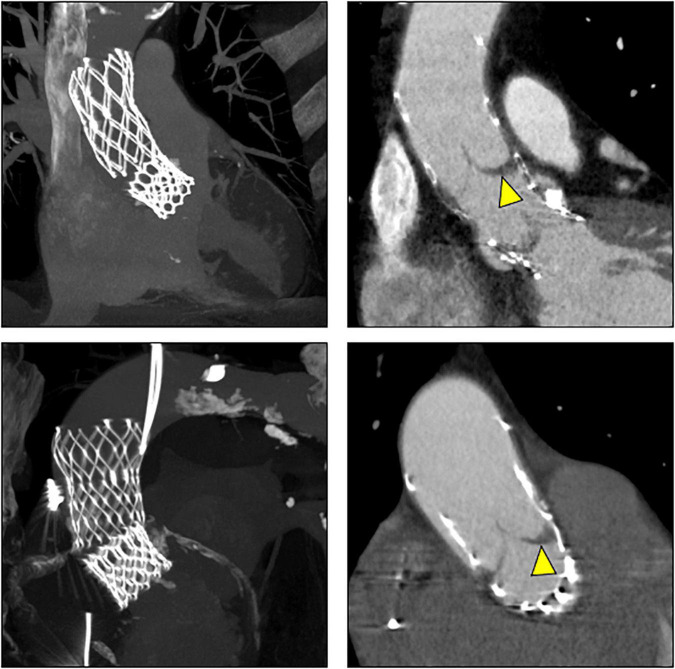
Subclinical valve thrombosis in embolized valves. In patients 9 (top) and 10 (bottom; see Table 5 for details), follow-up CT detected hypoattenuated leaflet thickening (arrow heads) in self-expanding valves embolized into the ascending aorta.
